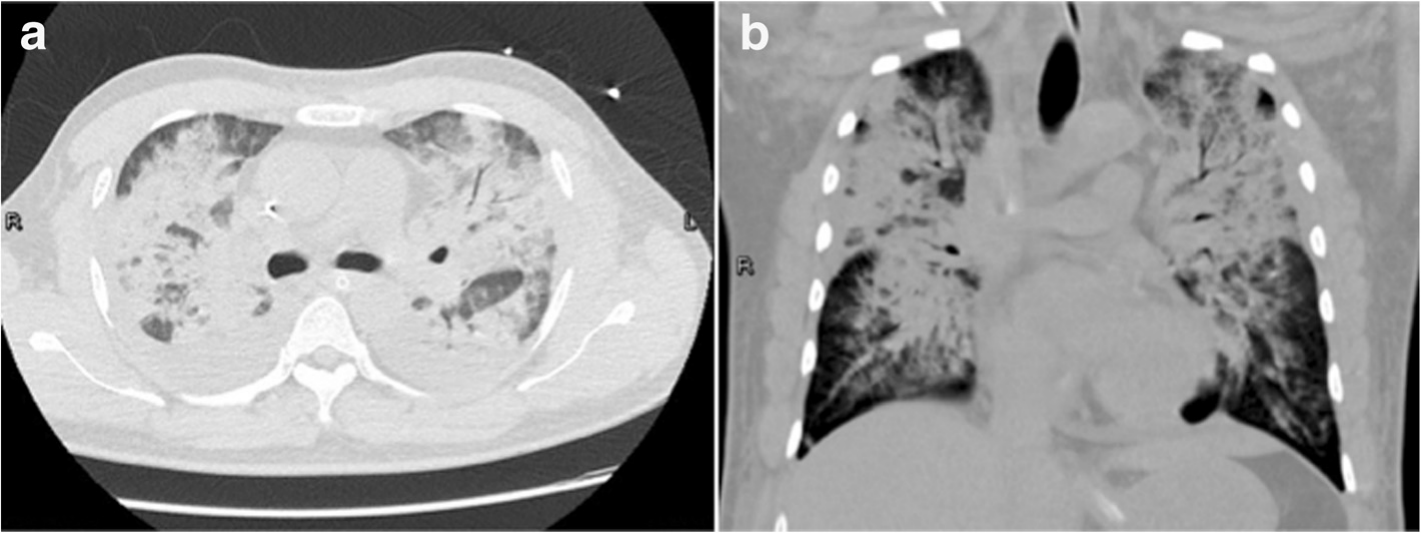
Chest CT showed diffuse air space lesions with air-bronchograms in both lungs (a) and (b)
